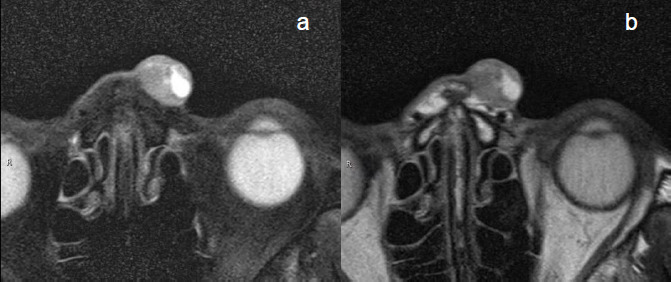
MRI of BCC in left nasal root showed hyperintense on T2WI fat suppression with clear profile (a); the tumor infiltrated into subcutaneous fat on T2WI (b). Cystic structures can be observed within the lesion.
